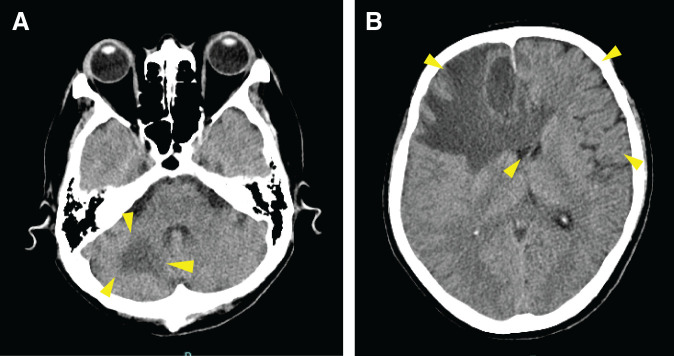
Postoperative cranial CT. (A) A ring enhancement in the right cerebellar hemisphere (yellow arrows). (B) Ring enhancement was also detected in the right frontal lobe (yellow arrows).
